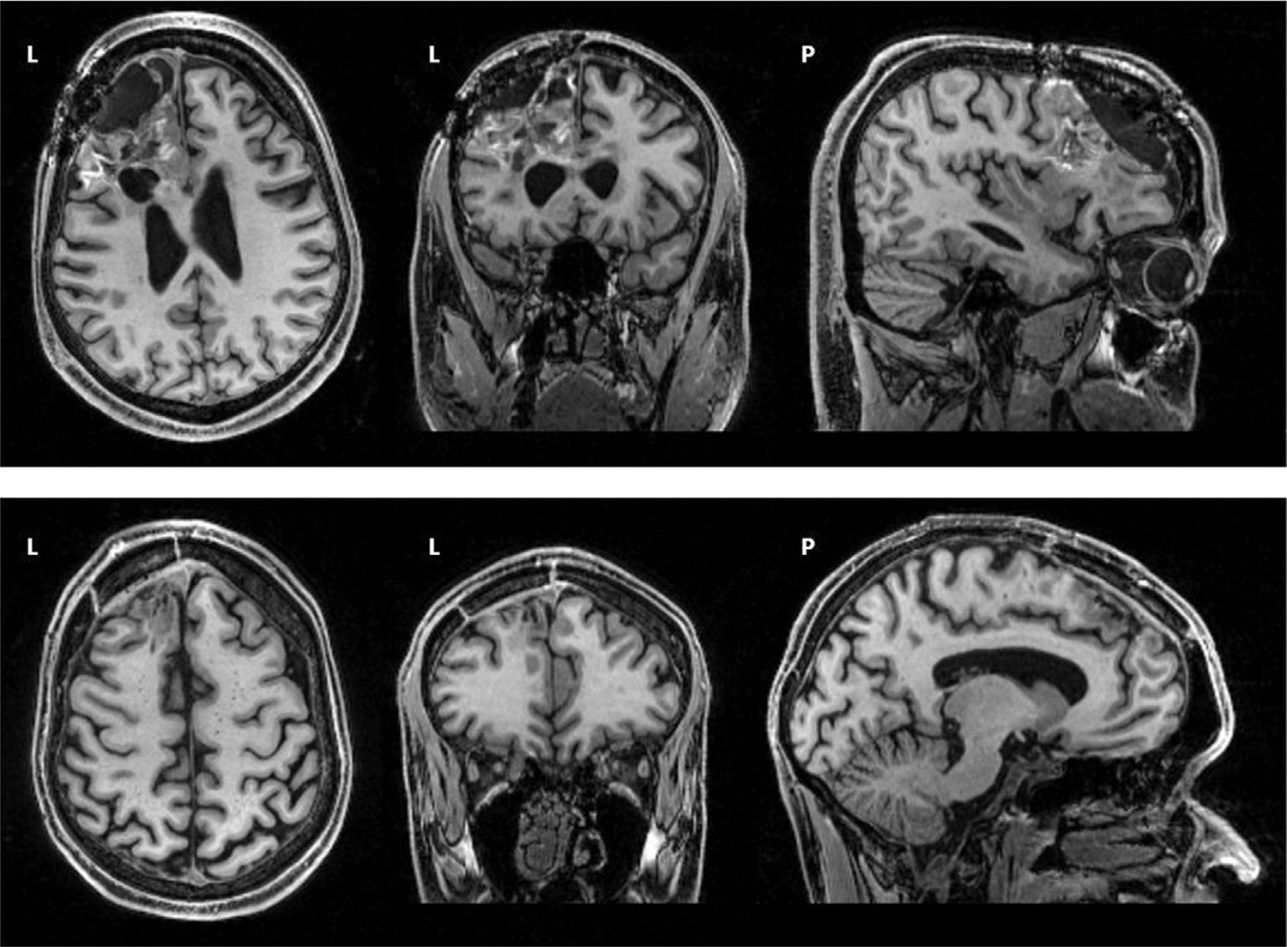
Examples of different frontal lesion severity. Top panel shows T1-weighted MR images from a patient with a severe/very severe frontal damage (total score=38). Bottom panel shows images from a patient with minimal/moderate damage (total score=6).
